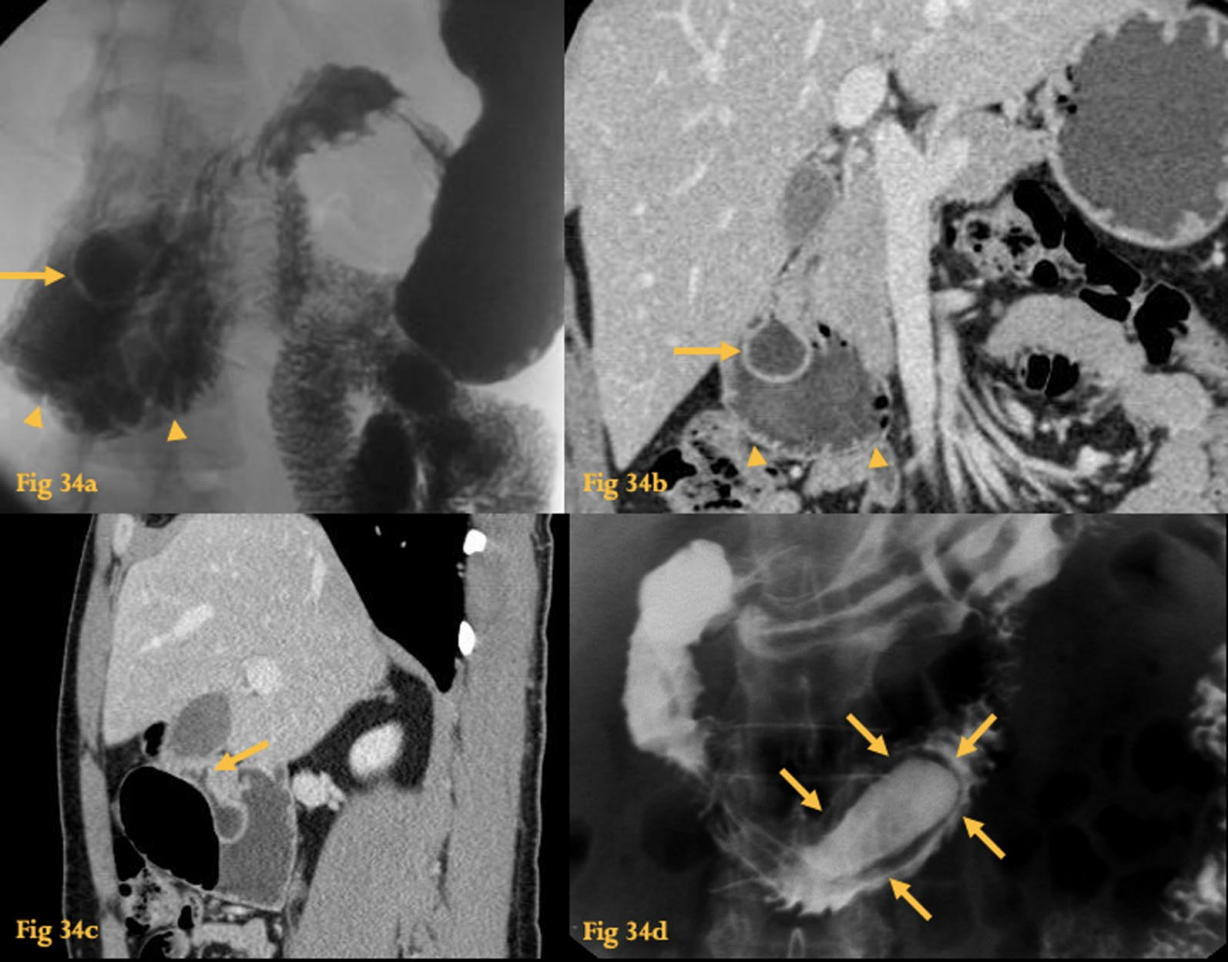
Intraluminal diverticulum. UGIS (a) shows a barium-filled sac surrounded by a radiolucent halo in D2 (arrow). Duodenal luminal dilatation and mucosal fold thickening are also noted (arrowheads). Abdominal CT (b) of the same patient with analogous findings. Sagittal reformation (c) clearly shows the attachment of the intraluminal diverticulum to the duodenal wall (arrowhead). UGIS of another patient (d) with an intraluminal diverticulum shows a radiolucent line surrounded by barium both inside and outside (arrows)
